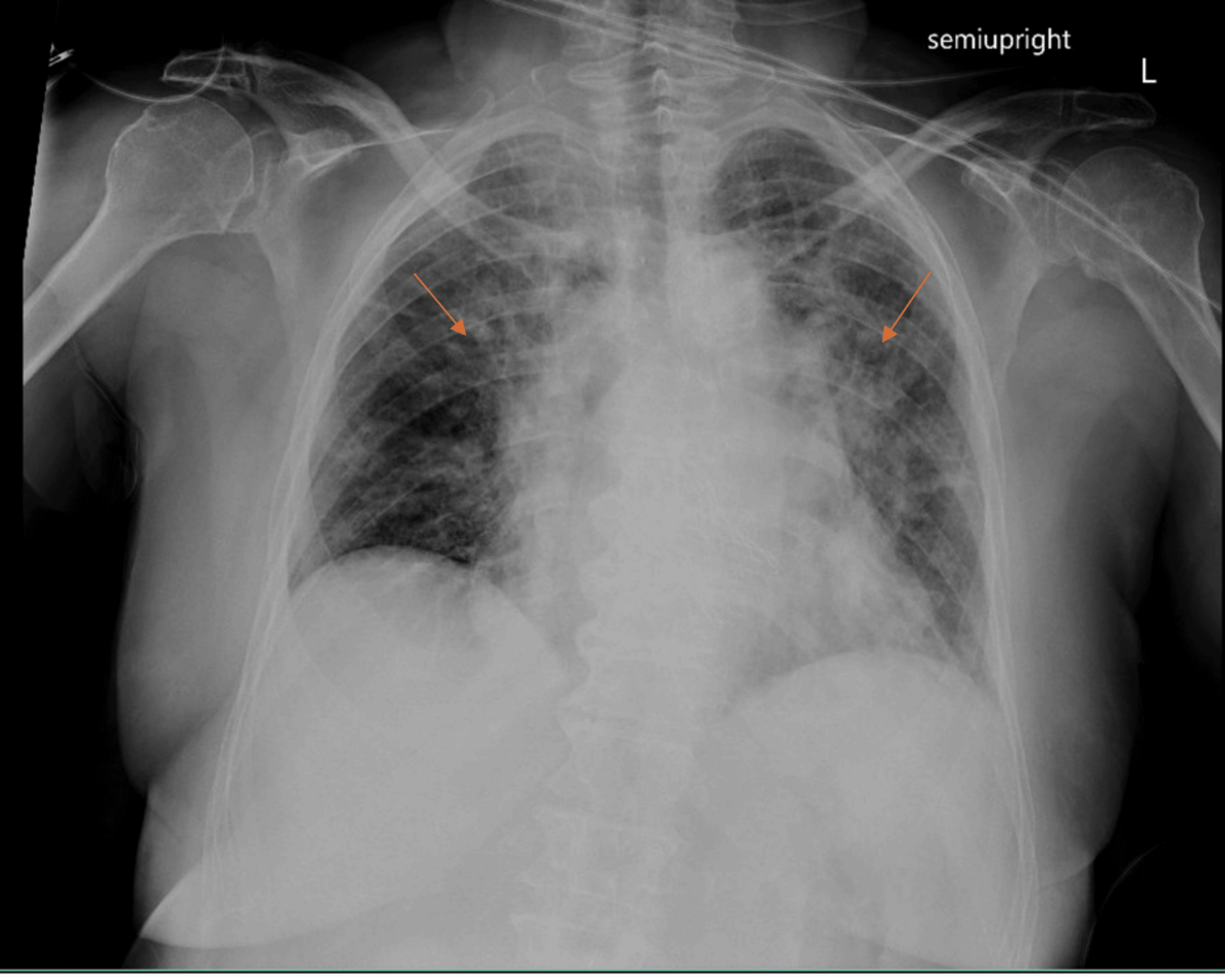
Chest X-ray following transcatheter aortic valve replacement (TAVR). Postsurgical changes from TAVR. Probable mild pulmonary edema/fluid overload (red arrows), accentuated by lung hypoinflation. This shows no acute finding to suggest this patient's constellation of symptoms.
